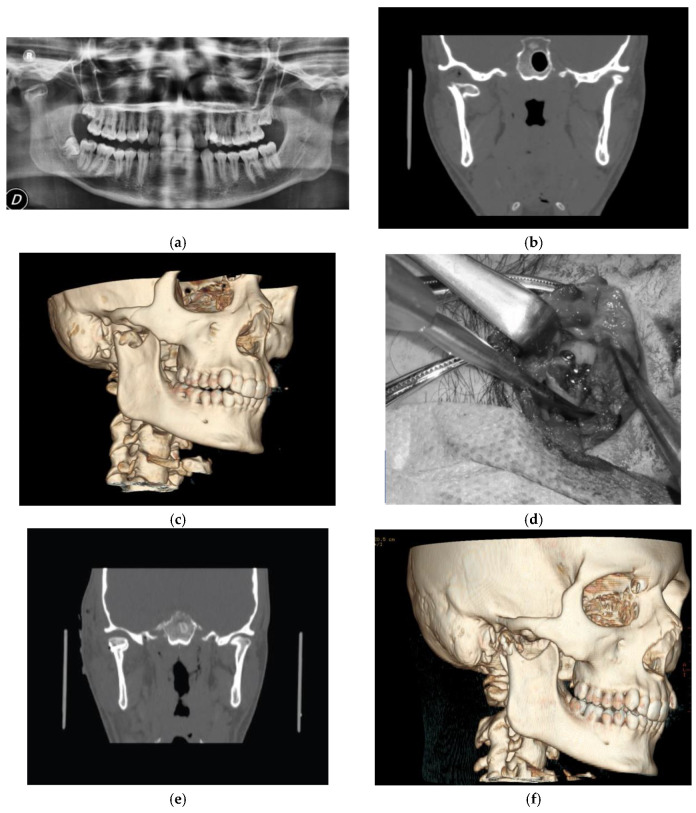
(a) Pre-operatory panoramic X-ray; (b) pre-operatory computerized tomography scan (CT scan); (c) pre-operatory volumetric CT scan; (d) intra-operatory view; (e) post-operatory coronal CT scan; and (f) post-operatory volumetric CT scan of patient #1.
